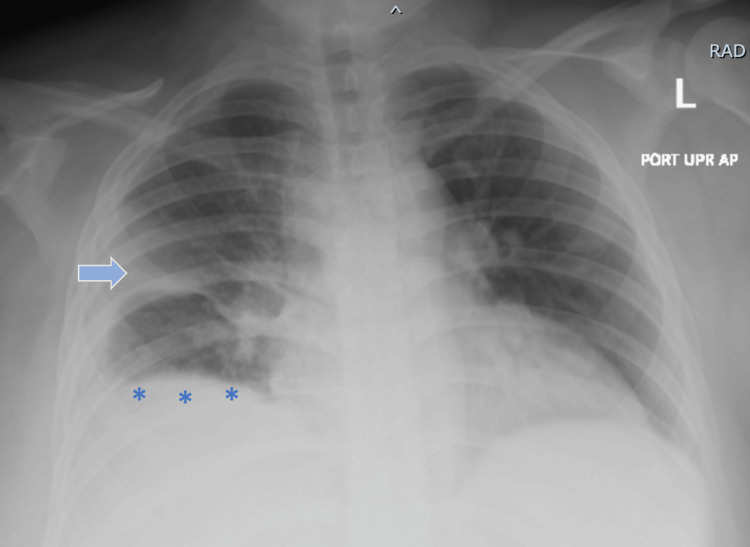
Chest X-ray findings Chest X-ray demonstrating right middle lobe infiltrate (blue arrow) and right-sided pleural effusion (asterisks)
